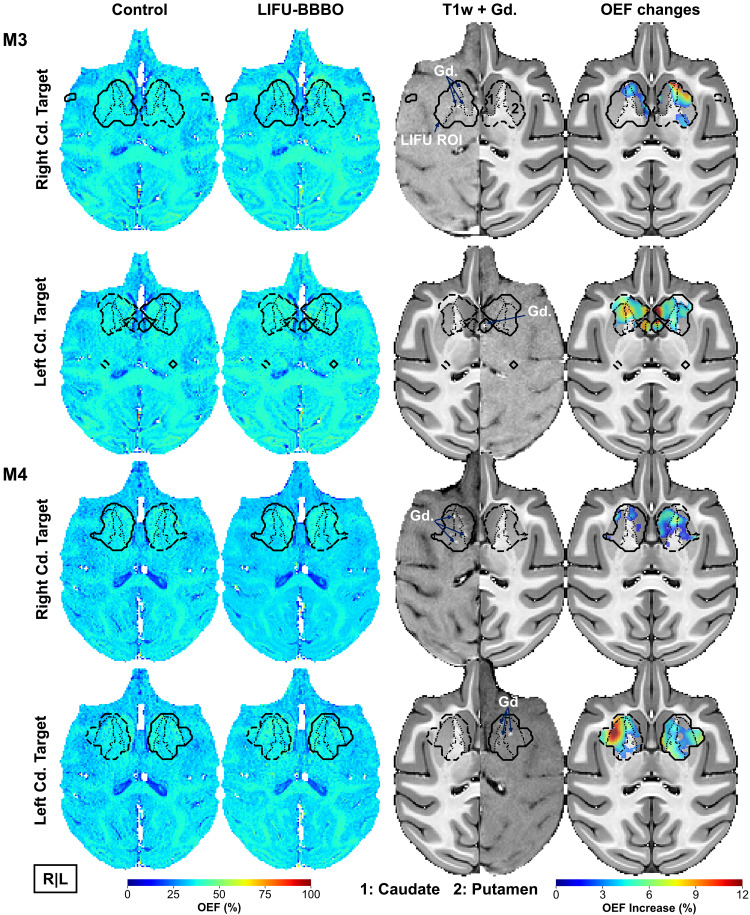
Representative OEF maps and corresponding anatomical images after LIFU-BBBO. Each row displays a different experimental session targeting either the right or left caudate nucleus (Cd. Target). The first two columns show OEF maps for control and LIFU-BBBO conditions, respectively. The third column shows postgadolinium (Gd) T1-weighted images, where dashed lines indicate the LIFU-BBBO ROI and solid lines delineate the caudate (1) and putamen (2). The final column displays the OEF change map, showing an increase in OEF primarily in the homologous contralateral striatum. Representative sessions from M3 and M4 are shown; slices are shown at consistent anatomical positions to facilitate comparison across sessions; note that these may not represent the location of maximum BBBO or peak OEF change for each individual session. Color overlays are thresholded to display only positive OEF changes for visualization clarity; complete unthresholded data including both increases and decreases were used in all statistical analyses. Quantitative volumetric analyses of all sessions are presented in Fig. 5 and Table 1.
